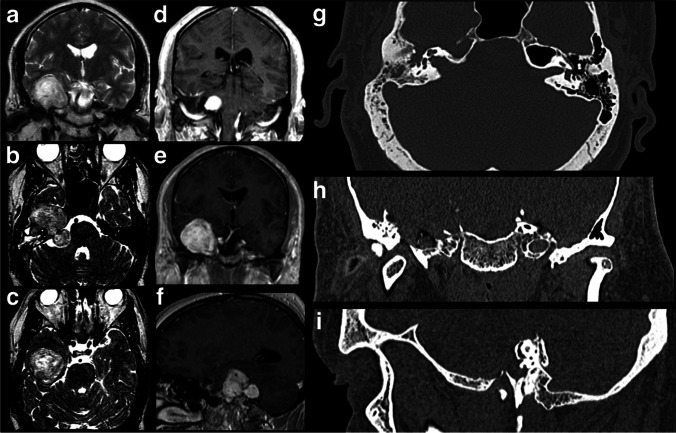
a–c Preoperative T2-weighted coronal and axial MRI cuts showing a relatively hyperintense heterogeneous well-circumscribed tumor, occupying the floor of the middle fossa, internal acoustic meatus, and posterior fossa CPA. It shows the classic dumbbell shape of a middle-posterior fossa facial nerves schwannoma. d–f Preoperative T1-weighted after gadolinium injection, showing the homogeneous enhancement of the different areas of the tumor. g–i Preoperative bone window CT scan axial, coronal, and sagittal cuts showing the huge erosion caused by the tumor in the middle fossa floor structures. The internal auditory meatus is widely enlarged by the tumor, while the petrous segment of the internal carotid artery is deprived of its bone cover
